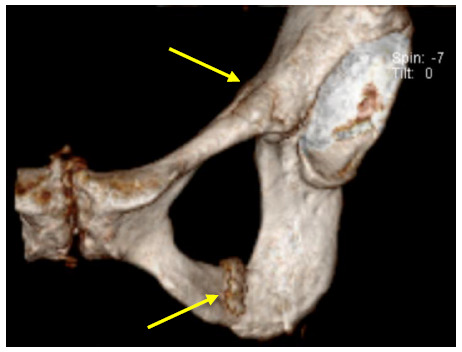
Case 4: 3-D CT scan of the pelvis and left hip four weeks after injury (top arrow showing acetabular fracture, bottom arrow showing ischial ramus fracture and callus formation)
